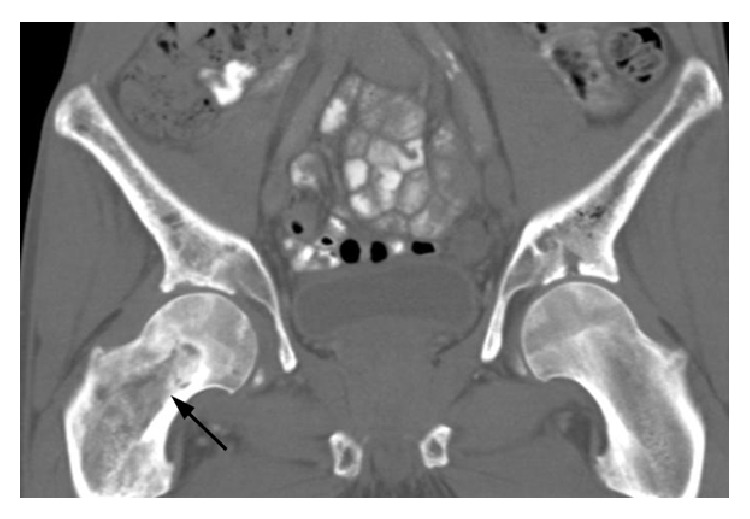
Coronal CT with oral contrast shows progression of bilateral femoral head osteonecrosis. There is also diffuse marrow signal abnormality of the visualized pelvis and right proximal femur (arrow), also believed to be consistent with osteonecrosis by imaging.
